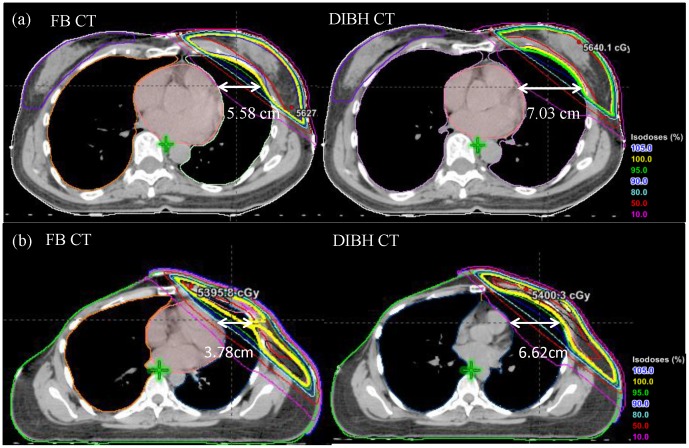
Anatomy and isodose comparisons for (a) the left breast and (b) the left CW patients (left: FB CT; right: DIBH CT). The CW expansion increased from 5.58
